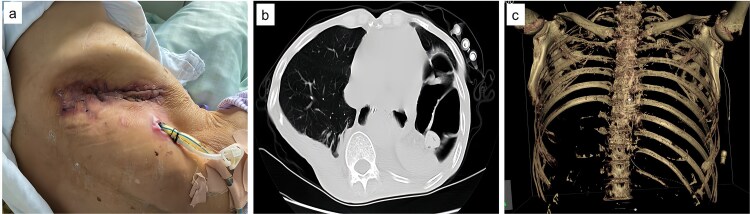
Postoperative images. (a) Fourteen days following the surgical procedure, the wound exhibited satisfactory healing. (b) One month after surgery, during the preparation for removal of the nasointestinal tube, a follow-up chest CT scan revealed that the abscess cavity had been completely filled. (c) Visual documentation of the removal of ribs 9th to 12th.
